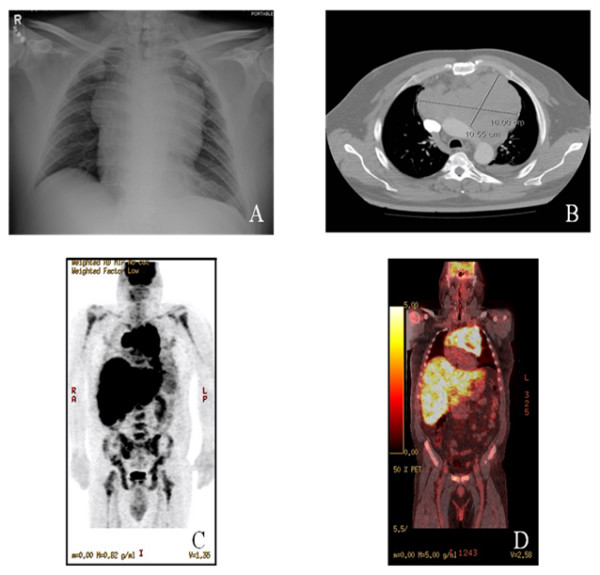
Chest X-ray, CT chest and PET/CT images. A. A chest x-ray (Frontal view) showing a huge mediastinal mass with evidence of mediastinal widening. B. CT scan of the chest showing 3 anterior mediastinal masses with the largest one being 16 cm × 10 cm. C. PET image revealing a very large anterior mediastinal mass and a diffuse liver involvement along with abnormal activity in both humeri, femura, and pelvic bones. D. Fusion PET/CT image showing a very large anterior mediastinal mass extending across the mediastinum from left to right and superiorly into the supraclavicular area. A diffuse involvement of the liver along with abnormal activity in both humeri, femura, and pelvic bones were also noticed.
